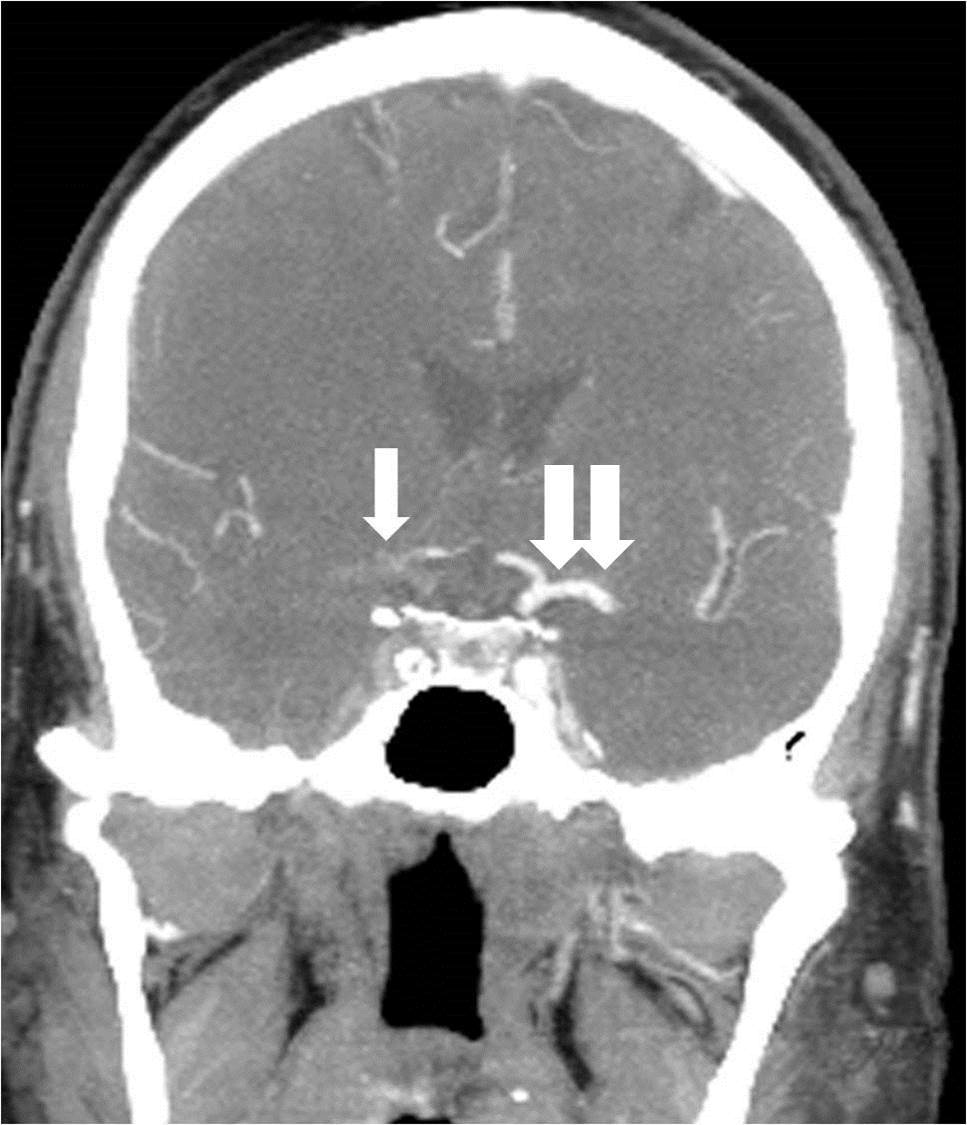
Coronal reconstruction from a computed tomography angiogram reveals a complete occlusion of the right middle cerebral artery and supraclinoid internal carotid artery (single arrow) Notice the normal contralateral middle cerebral artery and internal carotid artery (double arrow).
